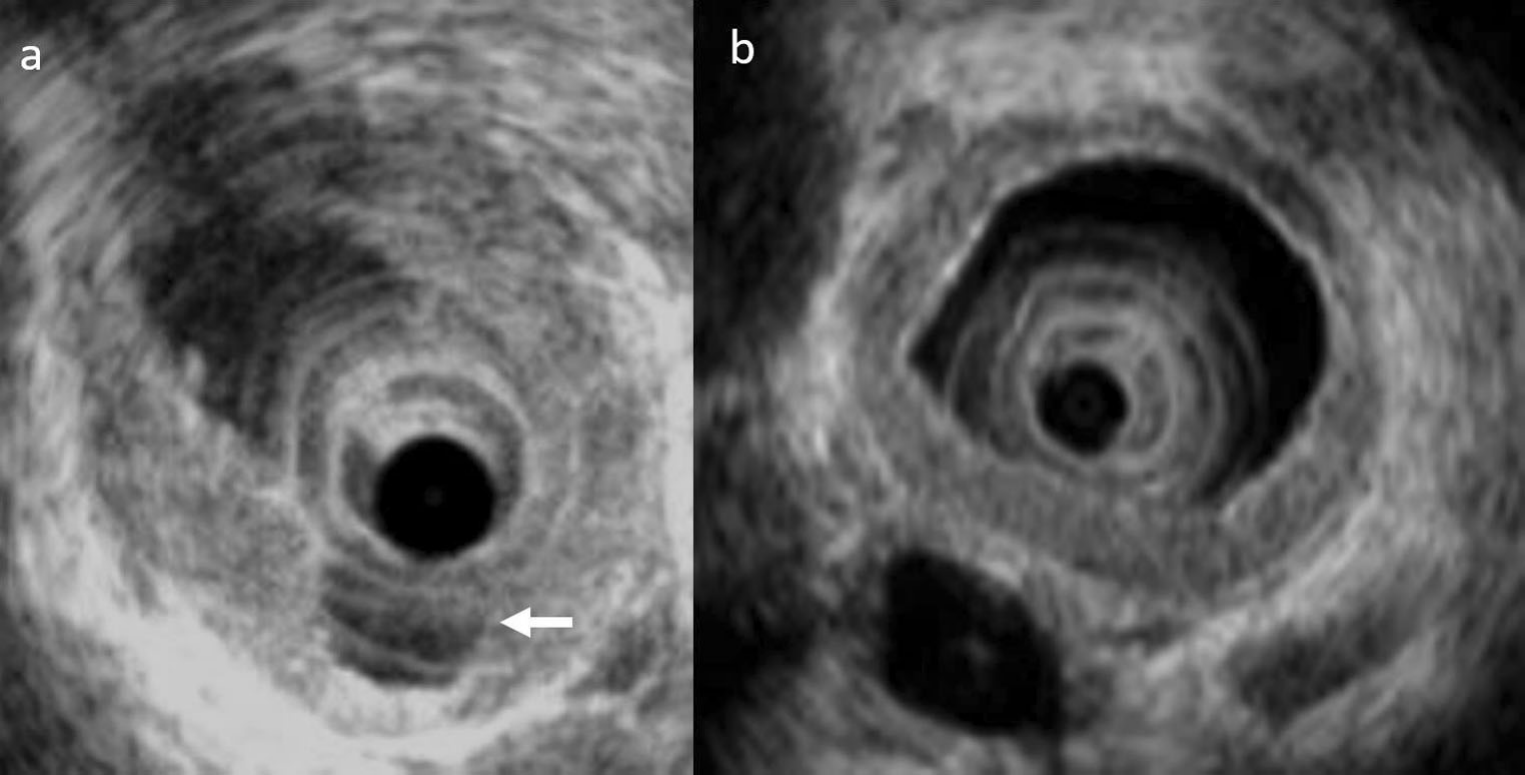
Intraductal ultrasound (IDUS) findings. a IDUS of PSC: this image shows an irregularity of the bile duct epithelium and diverticulum-like outpouching (white arrow). b IDUS of IgG4-related SC: bile duct epithelium is smooth and preserves three-layer structure. Regular hypoechoic thickening in the inner part can be seen
